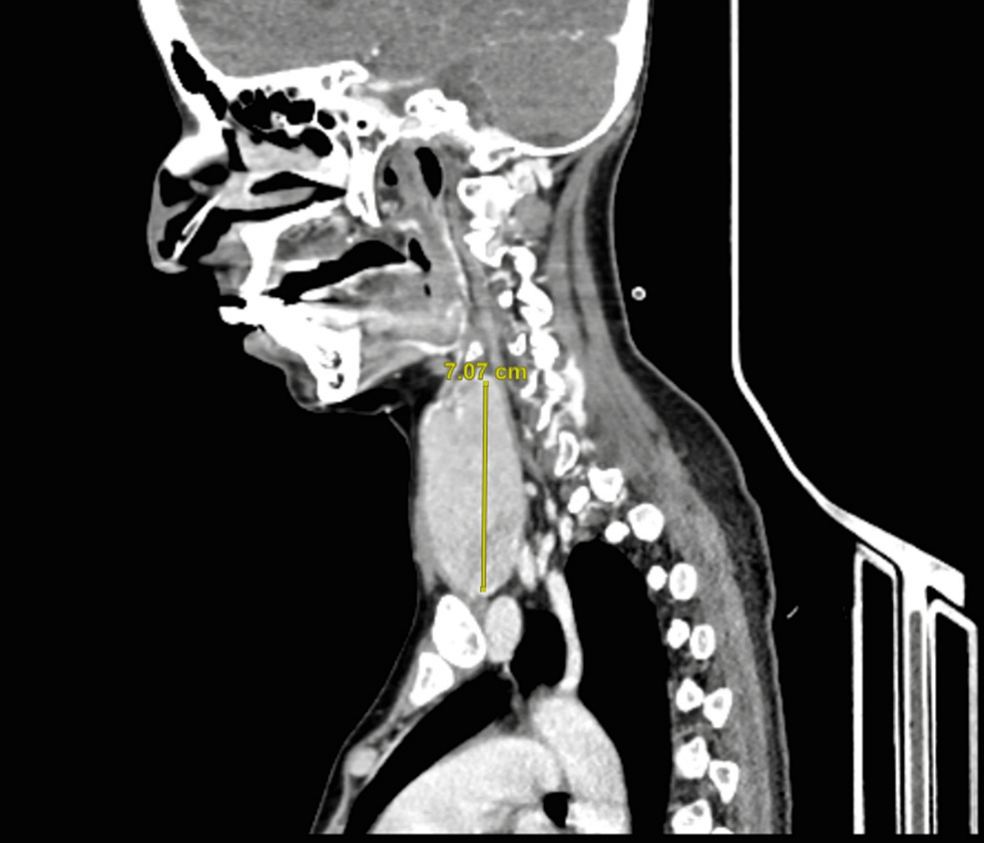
Neck CT (sagittal view) showing markedly enlarged thyroid gland with no retrosternal extension. The yellow line delineates the size of the thyroid gland. CT: computed tomography
